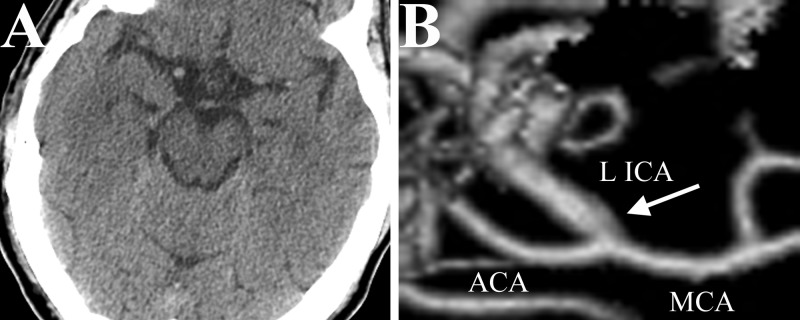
Baseline normal imaging studies A) Axial computed tomography (CT) of the brain without contrast showing no acute hemorrhage in basal cisterns. B) 3Dimensional reconstruction of CT angiography of the neck showing normal intracranial vessel anatomy, *white arrow* indicates left supraclinoidal internal carotid artery without evidence of aneurysm. ACA:* *anterior cerebral artery; LICA: left internal carotid artery; MCA: middle cerebral artery*.*
